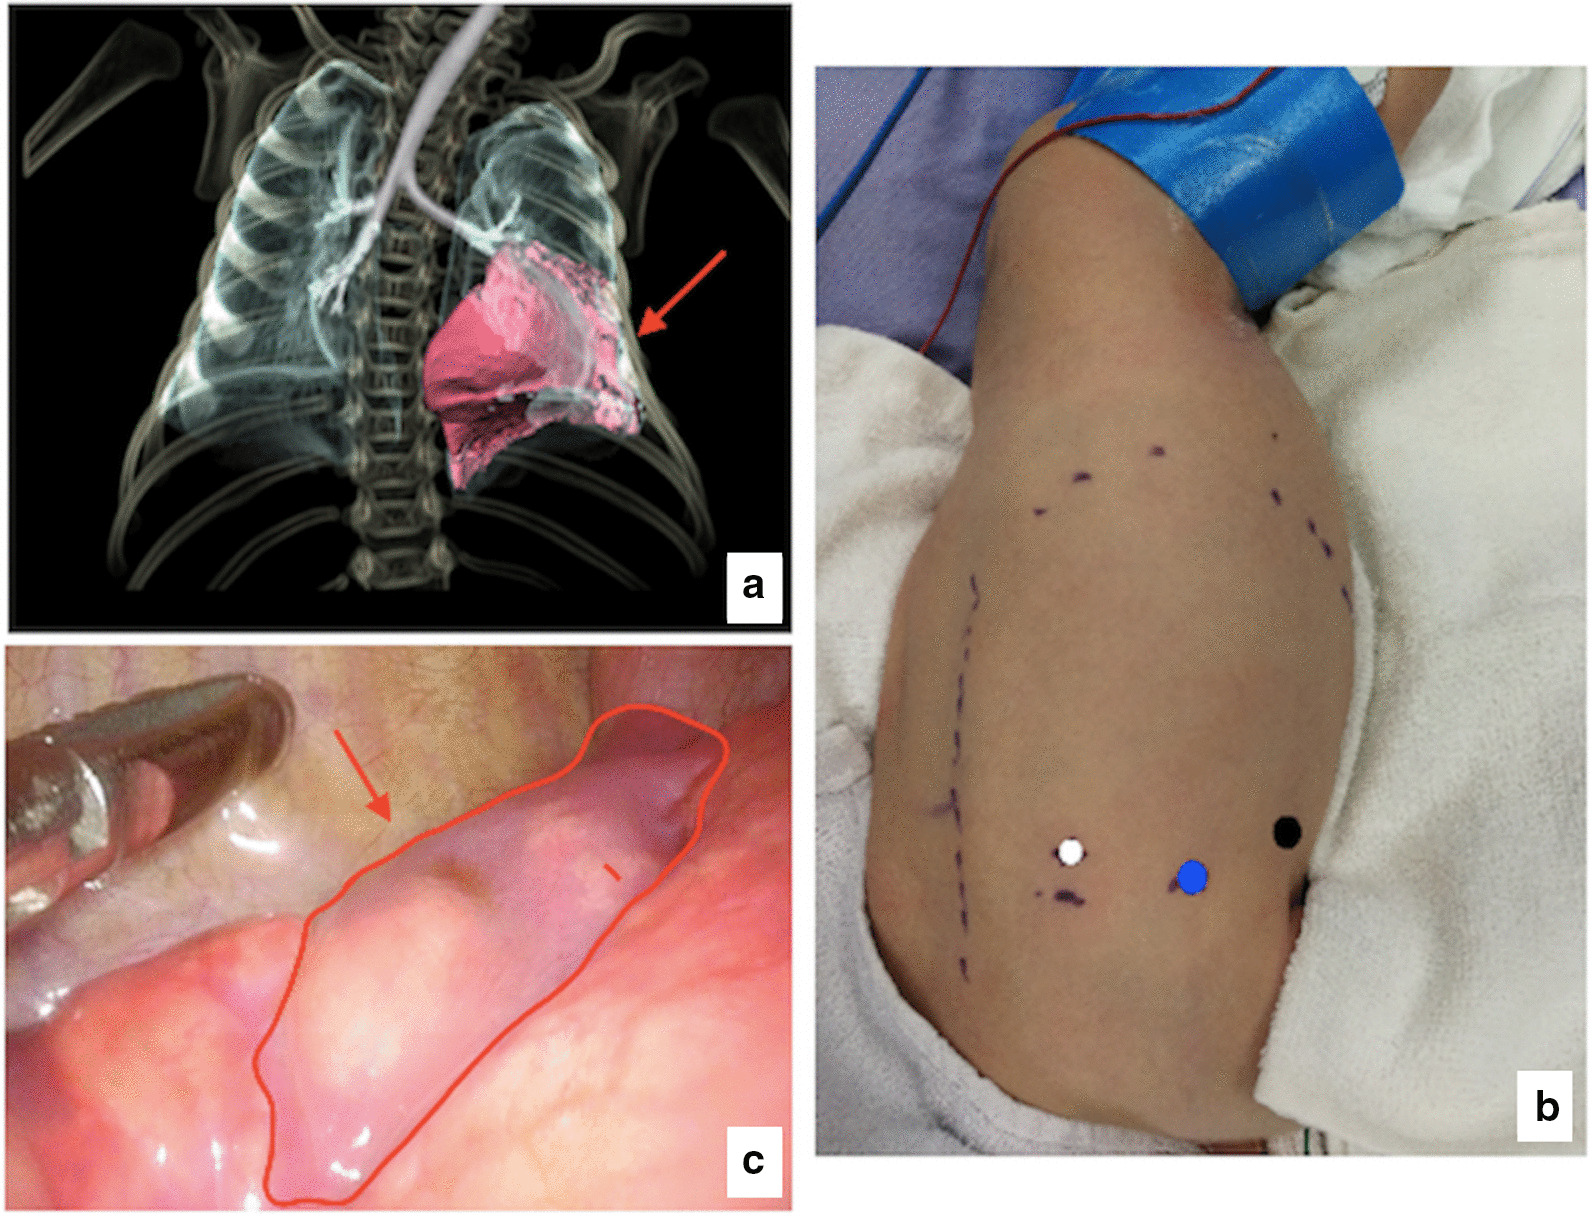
a Preoperative CT scan of a left lower lung segmental CPAM (arrows), cross-sectional view. b The patient was placed in a semiprone position with the affected side elevated as recommended for left thoracoscopic lower lesionectomy in a neonate (3-mm ports, white circles; a 5-mm port, blue circle, for camera; 5-mm port, black circle, for ultrasonically activated scalpel). c Intraoperative photograph of CPAM (arrows)
